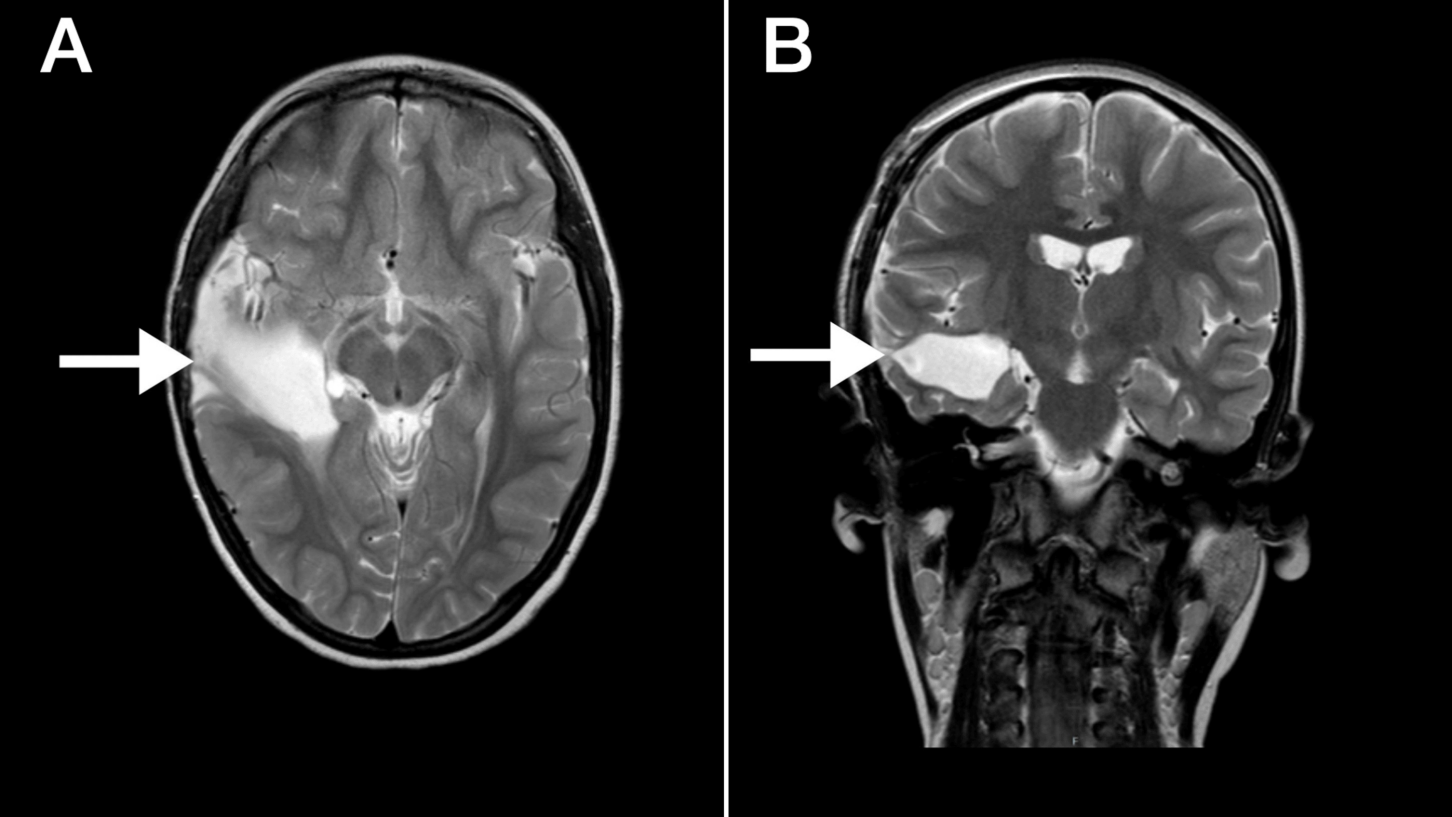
(A) Axial T2-weighted image demonstrates a hyperintense, fluid-filled cleft in the right temporal lobe communicating with the lateral ventricles (white arrow); (B) Coronal T2-weighted image confirms the fluid-filled cleft in the right temporal region communicating with the lateral ventricles (white arrow).
